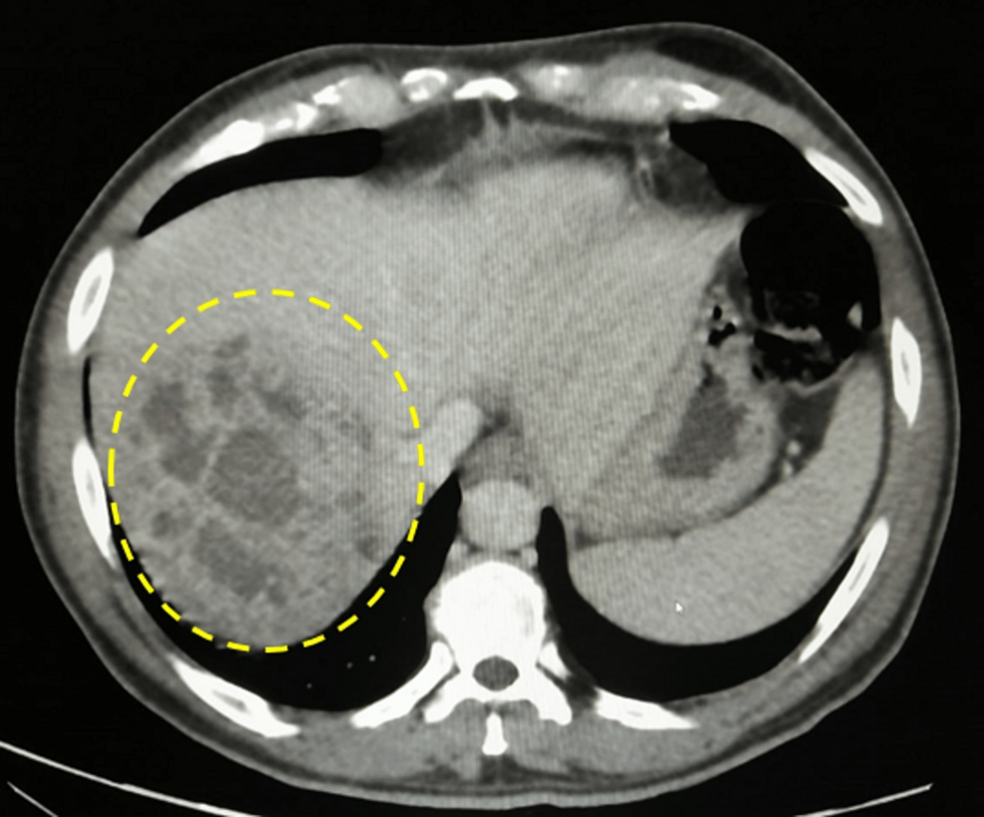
Abdominal CT scan The scan shows large multiloculated cystic lesions in segment 8/7 of the liver (dashed circle).
